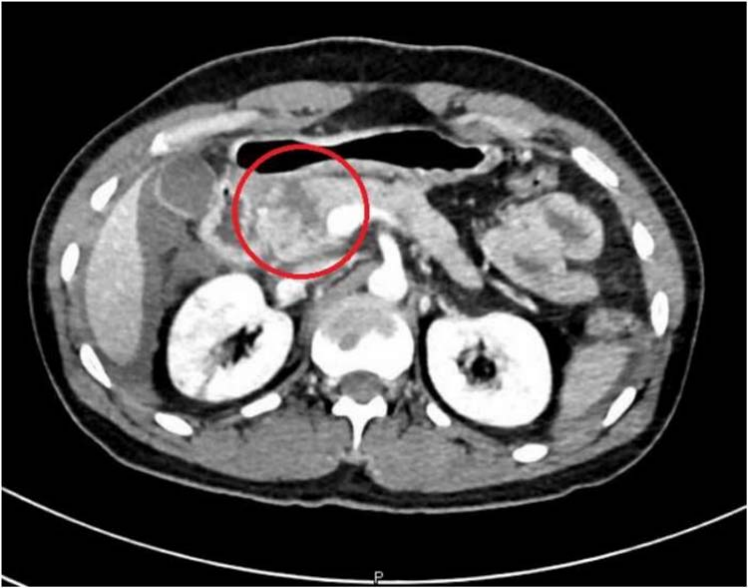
CT abdomen—sagittal section, showing transection of the pancreas (circle).
